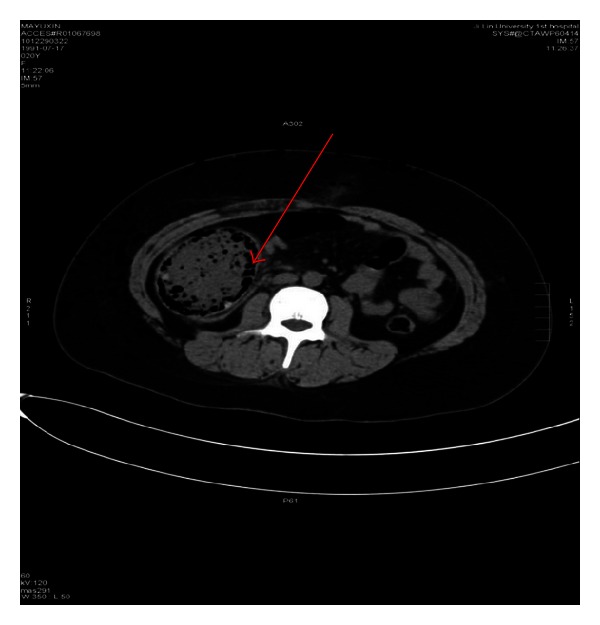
CT scan of abdomen shows distended ascending colon, contained fecal matter, and air entrapment (arrow) with postappendectomy changes.
